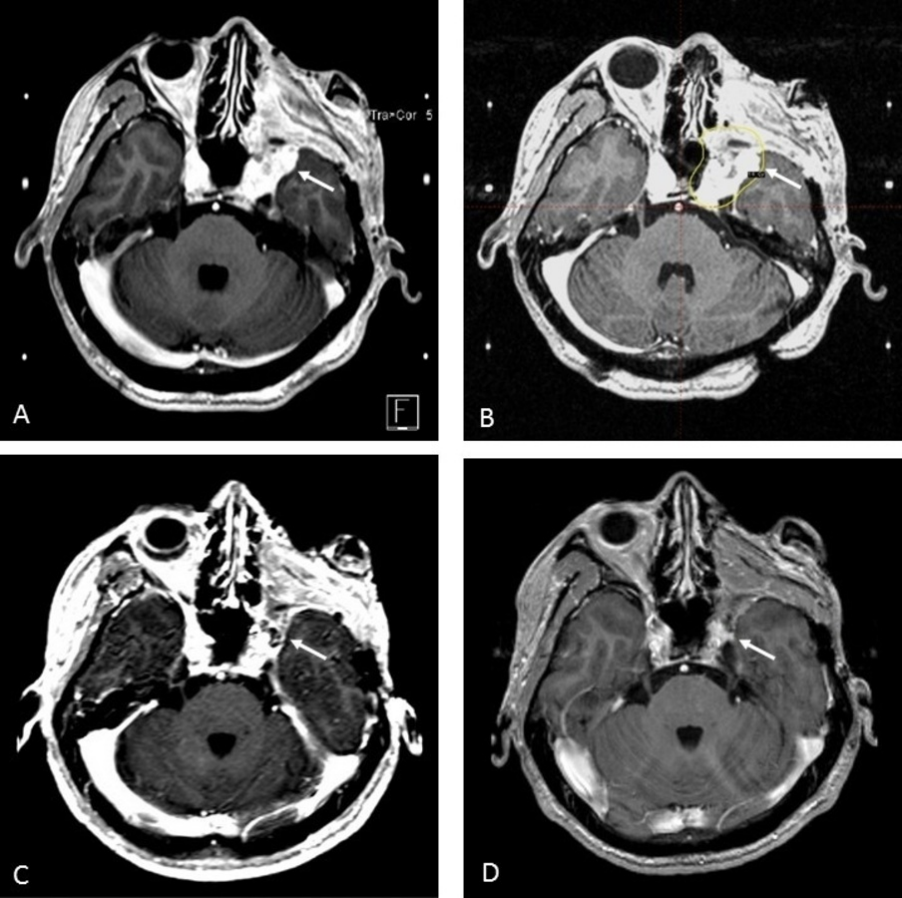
Stereotactic Radiosurgery Treatment for Recurrent Nasopharyngeal Carcinoma (A) Recurrent nasopharyngeal carcinoma in the left cavernous sinus (arrow); (B) Definitive stereotactic radiosurgery treatment plan. All gross disease covered by 14 Gy isodose line, prescribed to 50% (arrow); (C) Six-month follow-up MRI demonstrating decrease in size of soft tissue enhancement within left cavernous sinus; (D) Two-year follow up MRI showing persistent fullness in the left cavernous sinus region that has decreased in size from initial treatment and six-month scan.
